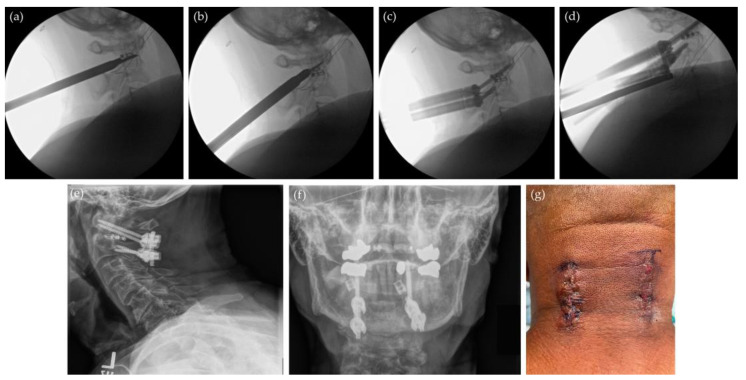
Minimally invasive modification of the Goel–Harms atlantoaxial fusion. Under fluoroscopic guidance and stereotactic navigation, (a) intraarticular cages are placed following joint preparation using a distractor. (b) The C1 lateral mass is then drilled within the potential space created by the cage placement. Subsequently, (c) C1 lateral mass and C2 pars screws with extended tabs are inserted. (d) Rods are inserted subfascially to complete the fixation. Postoperative (e) lateral and (f) anteroposterior radiographs show the implanted instrumentation and the intraarticular cages. (g) The procedure is performed via two paramedian skin incisions.
